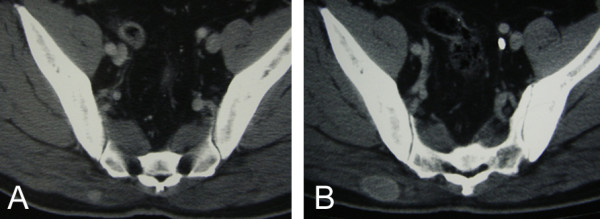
Computed tomography with contrast medium (100 ml, Omnipaque300, Daiichi-Sankyo, Japan) shows a well-defined mass in the gluteus maximus muscle, at the age of 60 years old (A; Toshiba, Aquilion^TM4^, 120 kV, 300 mA, 0.5 sec/r) and at the age of 65 years old (B; Toshiba, Aquilion^TM64^, 120 kV, 300 mA, 0.5 sec/r).
